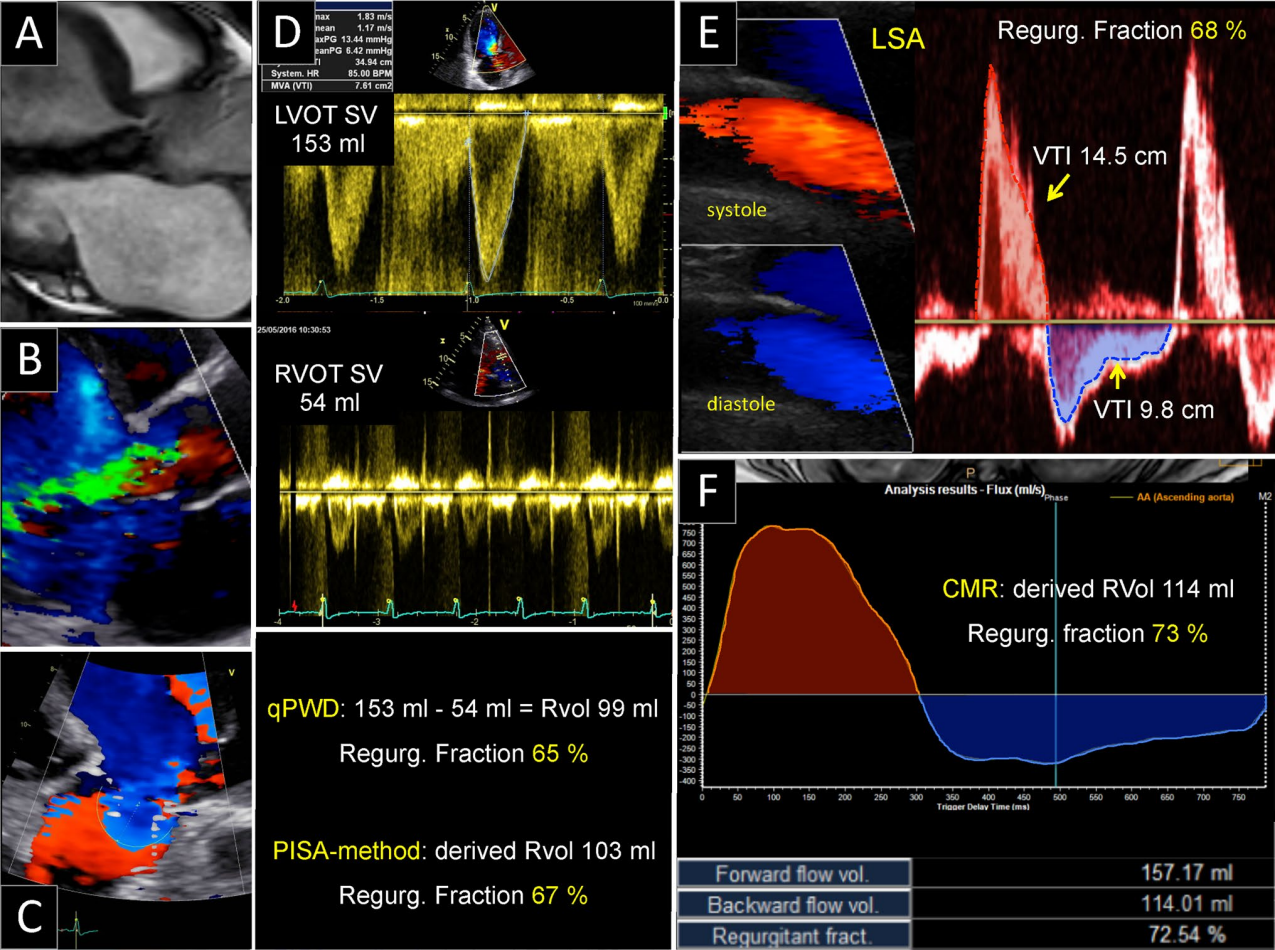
Example of a case with severe aortic regurgitation (AR). C Color Doppler depicting the flow convergence region of the AR from an apical log axis. Other references and abbreviations as in Fig. 1
